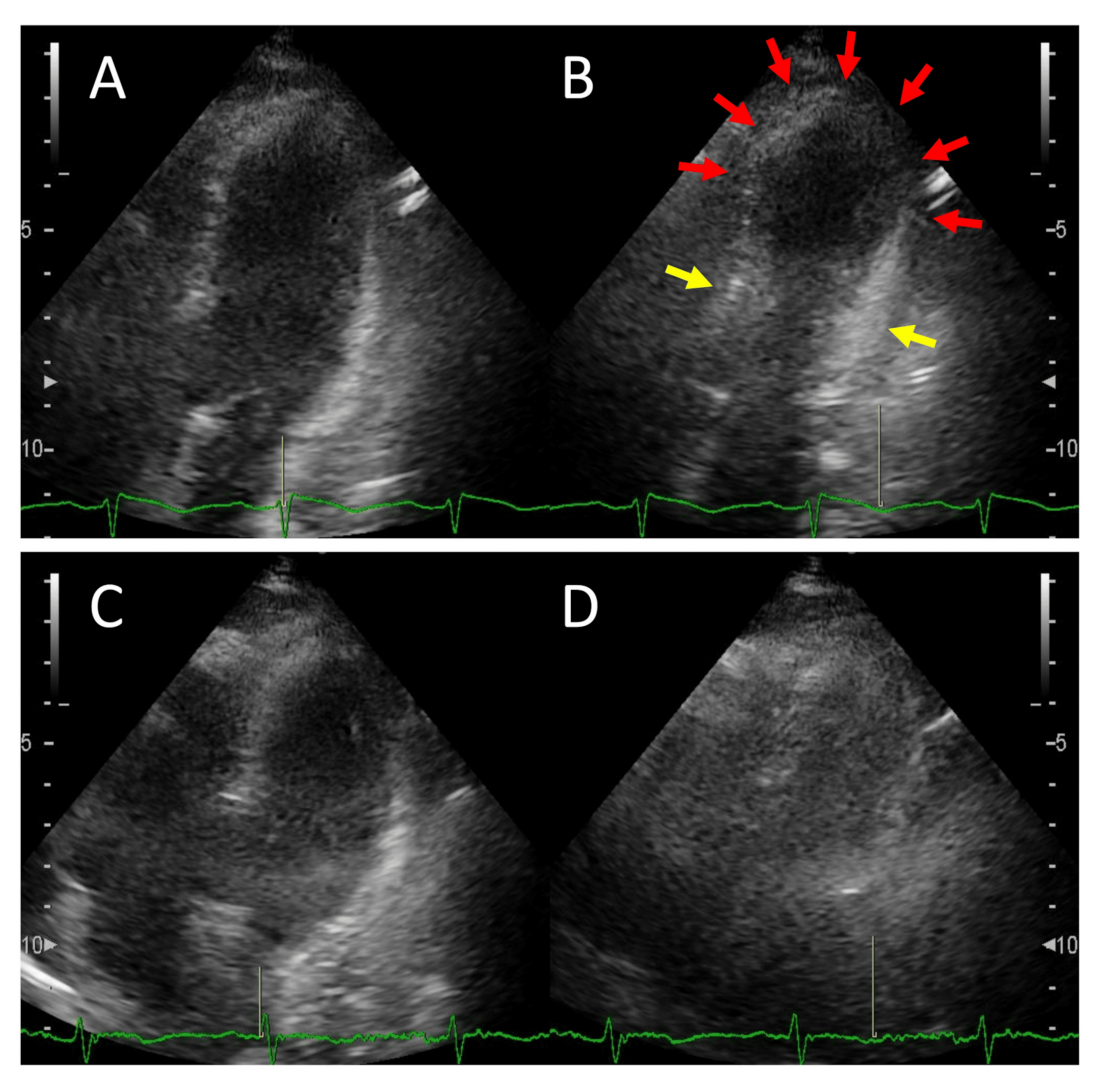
TTE findings at the onset of TTC and recovery period. Apical two-chamber view of TTE at the onset of TTC during diastole (A) and systole (B) showed left ventricular apical akinesia (red arrows) and preserved basal contraction (yellow arrows). TTE at the recovery period of TTC during diastole (C) and systole (D) demonstrated improved apical contraction. TTE: transthoracic echocardiogram; TTC: Takotsubo cardiomyopathy
